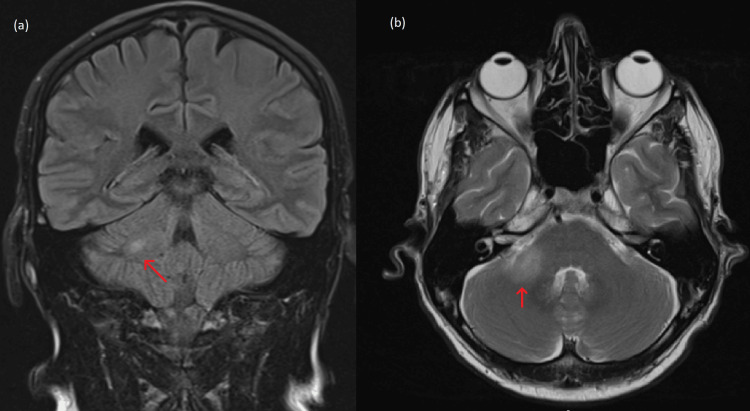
MRI whole brain. (a) FLAIR coronal sequence. (b) T2 post contrast axial sequence. Magnetic resonance imaging (MRI) of whole brain showing enhancement in the cerebellar peduncle. (a) Selected section through the right middle cerebellar peduncle showing faint oedema. (b) Selected section though the cerebellar peduncle showing an enhancing right nodule indicating a second focus of rhombencephalitis. FLAIR: fluid-attenuated inversion recovery
